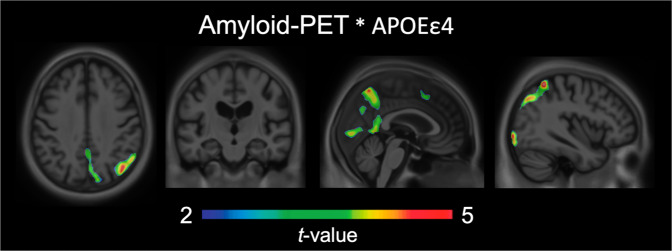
Cerebral tau aggregation depends on the synergistic interaction between amyloid-β and APOEε4. The synergistic interaction between [^18^F]AZD4694 and *APOEε4* carriership was related to increased [^18^F]MK6240 uptake in the posterior cingulate, precuneus, occipital, and inferior parietal cortices. T-statistical parametric maps were corrected for multiple comparisons using a Random Field Theory cluster threshold of *P* < 0.005, overlaid on the ADNI reference template. Age, clinical diagnosis, and amyloid-β SUVR were employed as covariates the model.
